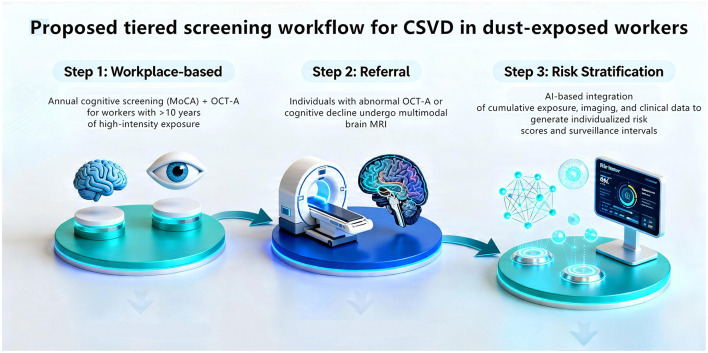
A three-step screening algorithm for occupational settings. Step 1 (workplace-based annual screening): cognitive assessment (MoCA) and OCT-A for workers with >10 years of high-intensity exposure. Step 2 (referral to occupational health center): abnormal OCT-A or MoCA triggers multimodal brain MRI (WMH volumetry, microbleed detection). Step 3 (AI-based risk stratification): integration of cumulative exposure, imaging, and clinical data to generate individualized risk scores and surveillance intervals. MoCA, montreal cognitive assessment; OCT-A, optical coherence tomography angiography; MRI, magnetic resonance imaging; WMH, white matter hyperintensities.
